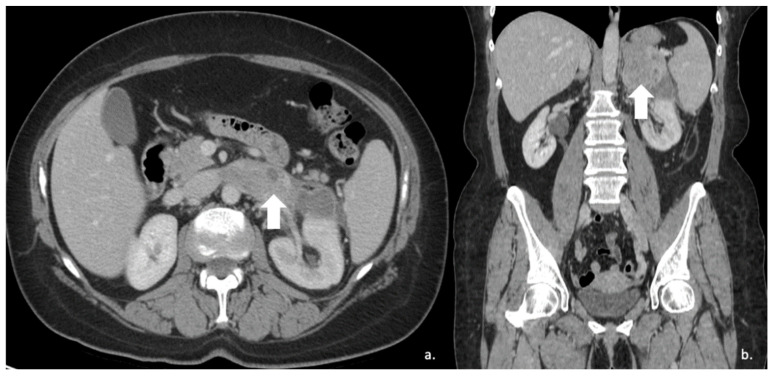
(a) Axial and (b) coronal venous phases CT show an ACC of the left adrenal gland presenting as a large mass (arrow) of heterogeneous appearance with extensive neoplastic thrombosis of the ipsilateral renal vein.
